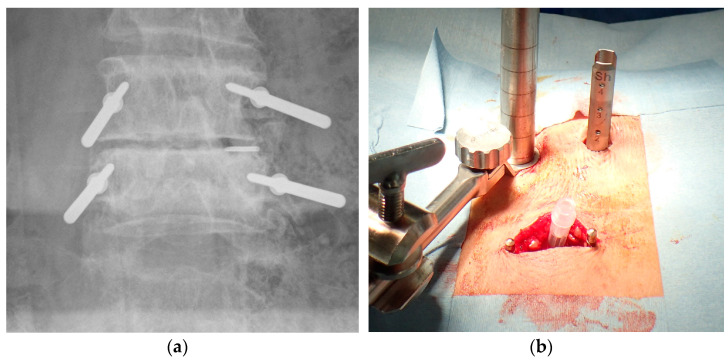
(a) Posteroanterior radiograph showing direction of self-drilling pins; (b) Pedicle screw insertion into the opposite side of the laminectomy via the tubular retractor.
